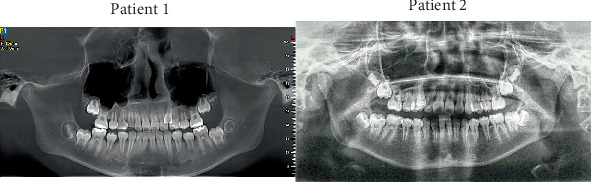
Pretreatment panoramic radiographs.
